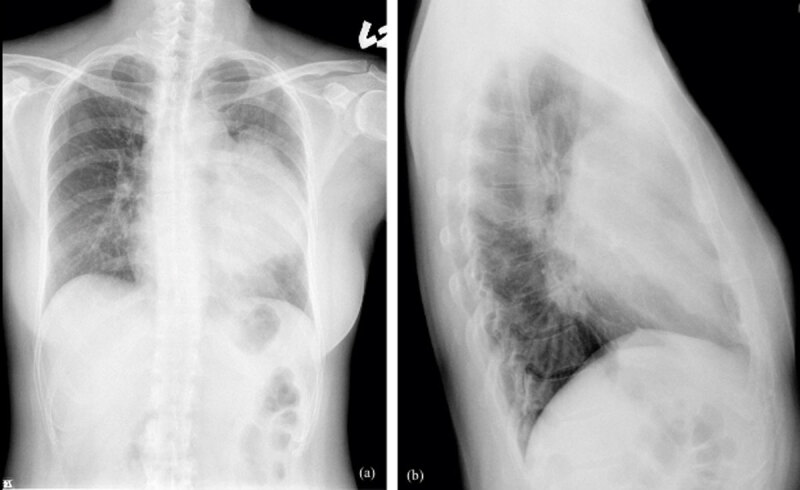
Chest radiographs PA (a) and lateral (b) views show a large anterior mediastinal mass lesion.
